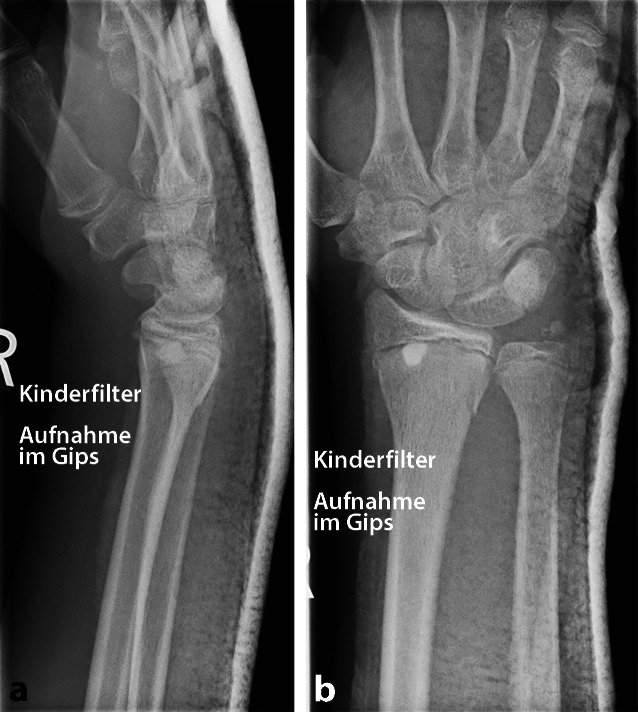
Das in unserer Notaufnahme durchgeführte CT erhärtete die Verdachtsdiagnose eines intraossären Fremdkörpers und zeigte einen zweiten kleineren intraossären Fremdkörper direkt volar des ersten (Abb. 3a, b).
